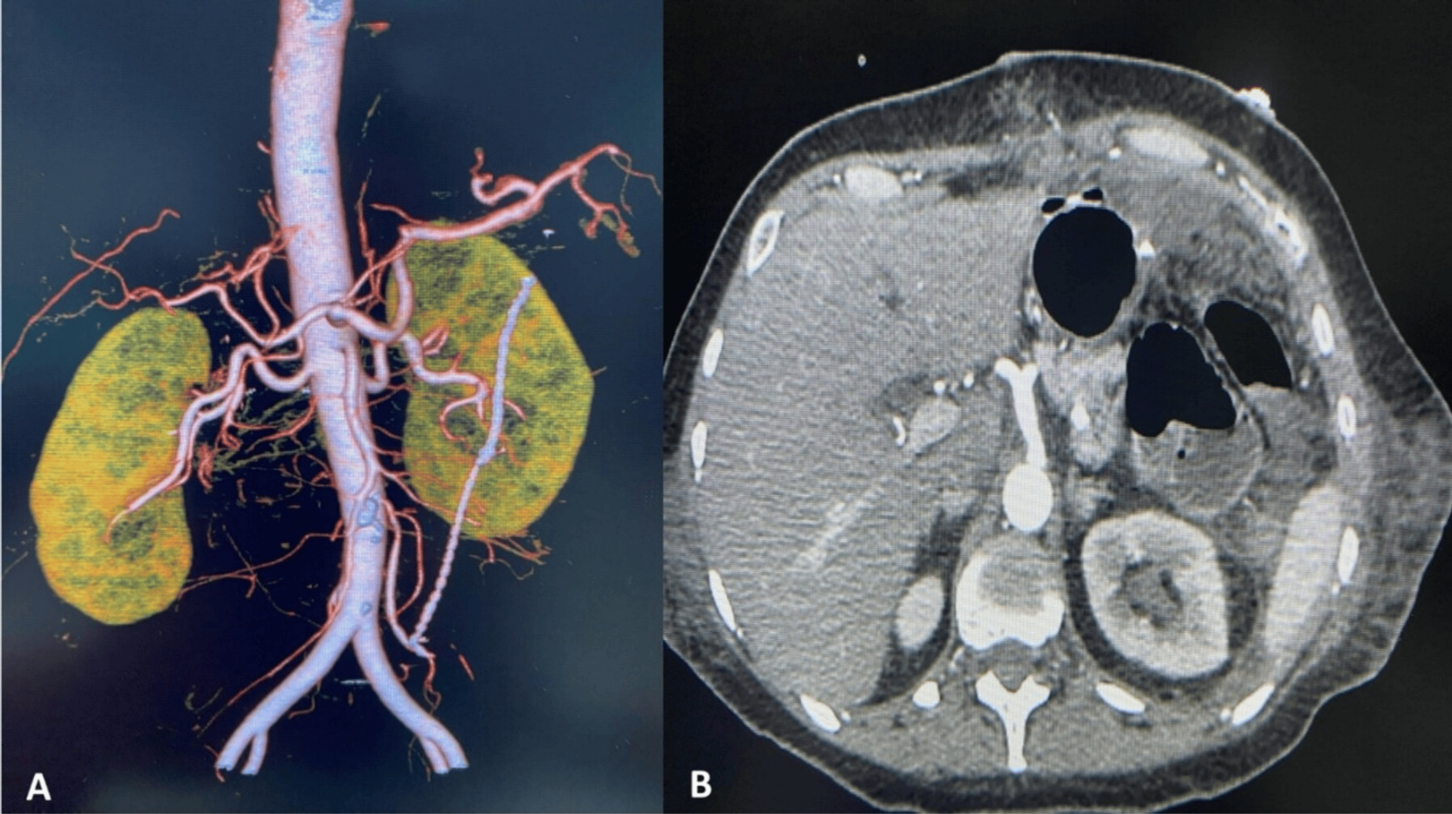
Axial and sagittal CT images of acute gastric necrosis with clear demarcation. (A) Abdominal CT angiography does not show vascular alterations in the abdominal trunks. (B) Axial tomography angiography shows integrity of the celiac trunk.
